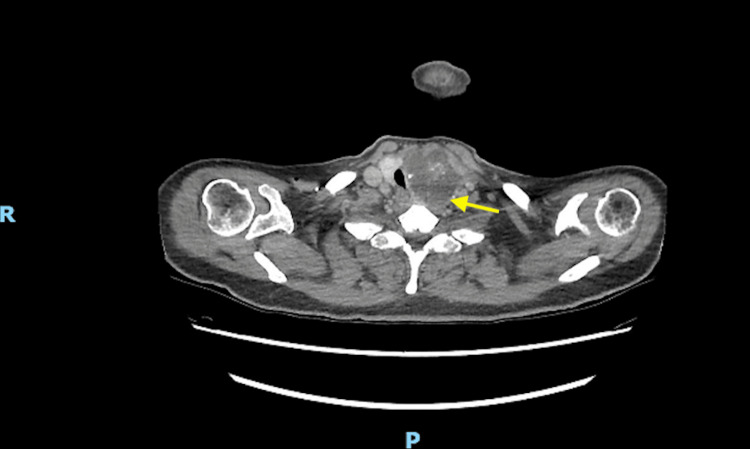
Axial view of the thoracic computed tomography scan showing large, lobulated, irregular, multiloculated mixed cystic/solid left thyroid mass with calcification, extending retrosternally.
